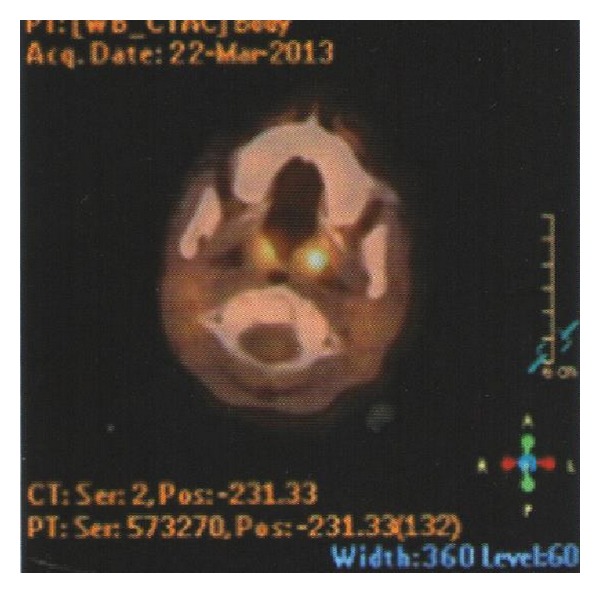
FDG-PET/CT, cervical lymph nodes.
